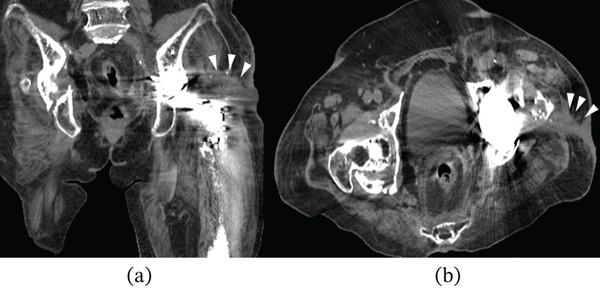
Preoperative computed tomography images showing the abscess formed around the proximal femur: (a) horizontal section and (b) axial section. Note the extensive osteolytic changes in the proximal lateral region of the femur (arrowheads).
